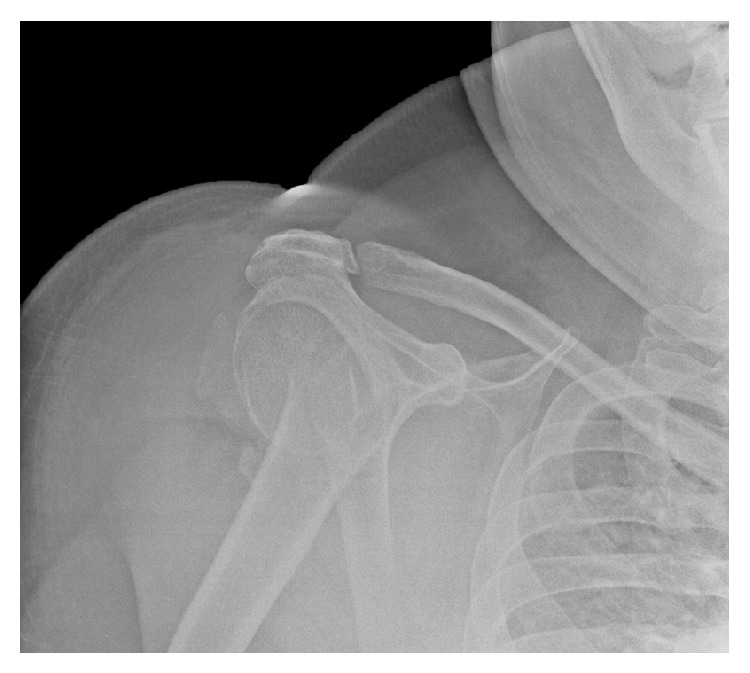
Radiograph of the patient's shoulder after the initial injury.
